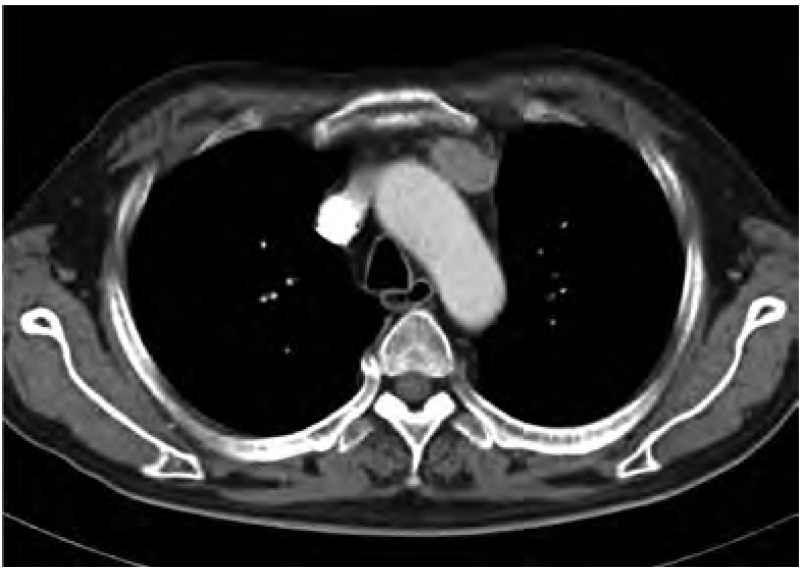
增强CT：可见胸腺类圆形病变，边界较光整，胸片上容易被主动脉弓及中轴骨的阴影遮挡。 Contrast-enhanced CT: Oval mass in thymic area with a clear boundary, which might be overlapping by opaque of aortic arch and axial bones in chest X-ray.
